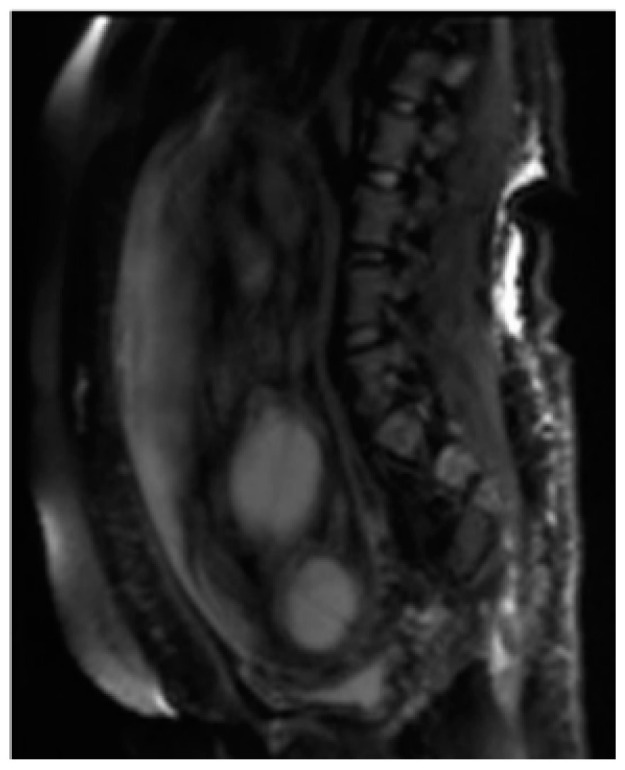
Diffusion Weighted Imaging (DWI).
